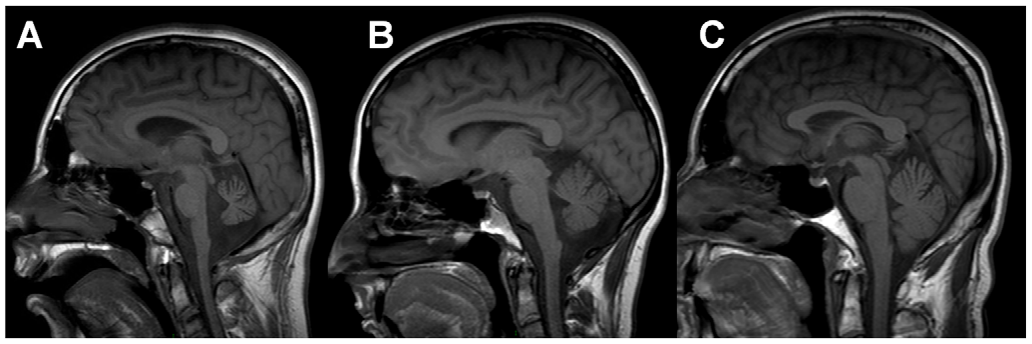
Neuroimaging of family members. T1 sagittal magnetic resonance imaging of the brain of IV-2 (A) reveals notable cerebellar atrophy. His asymptomatic mother, III-2 (B), has mild cerebellar atrophy, while his asymptomatic father, III-1 (C) has normal imaging.
